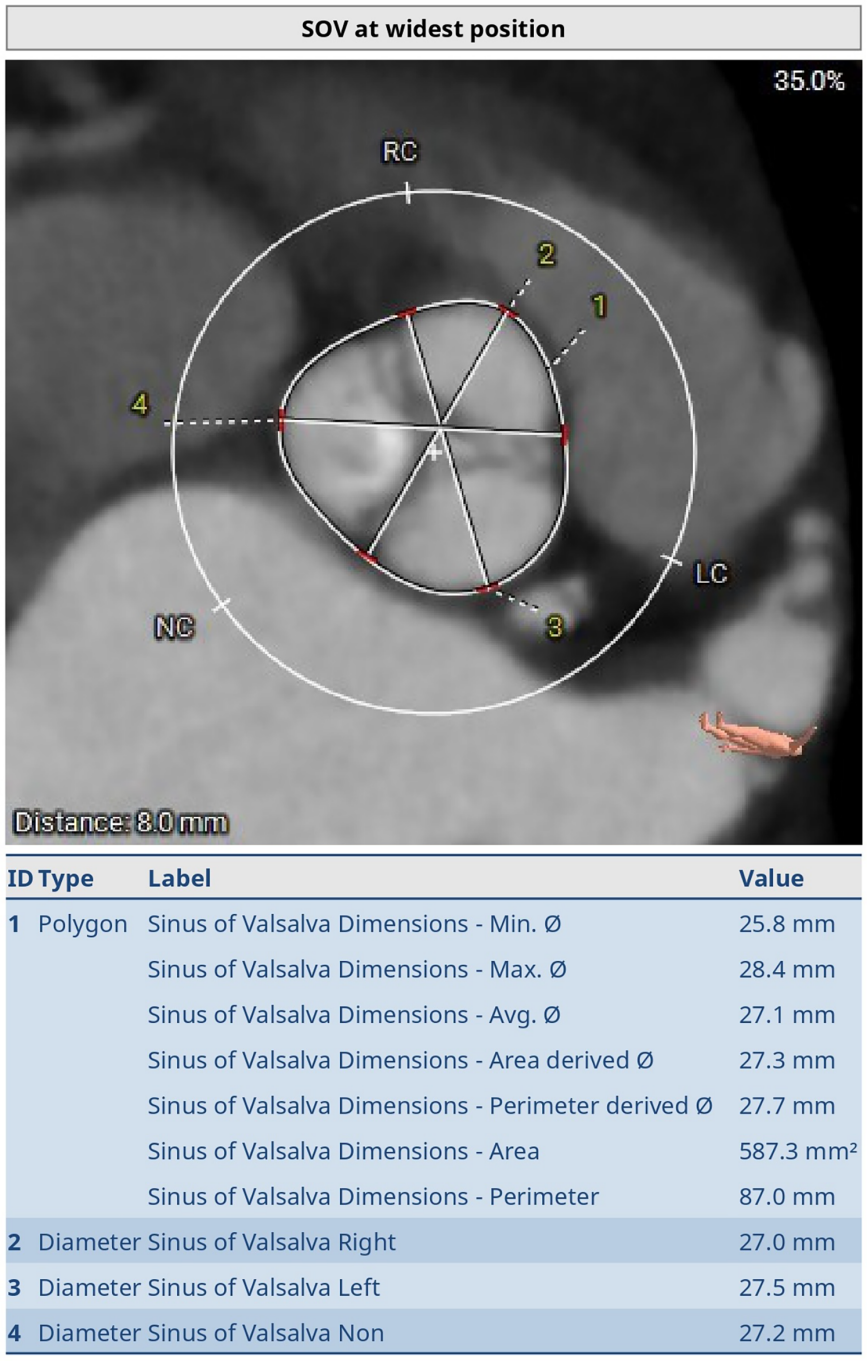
Preoperative thoraco-abdominal CT image showing the measurements of sinus of Valsalva (SoV) at the widest position. The SoV perimeter was 87.0 mm (minor SoV diameter 25.8 mm, major SoV diameter 21.1 mm) and the area was 587.3 mm^2^. Diameters of the right SoV, left SoV, and non-coronary SoV were 27.0 mm, 27.5 mm, and 27.2 mm respectively.
